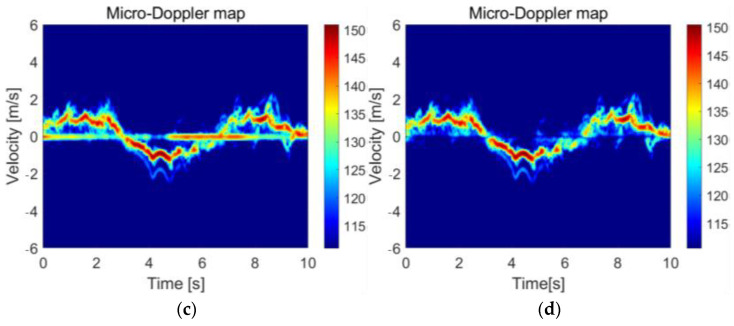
Range–Doppler heatmap: (a) before MTI and (b) after MTI. Micro-Doppler spectrogram: (c) before MTI and (d) after MTI.
